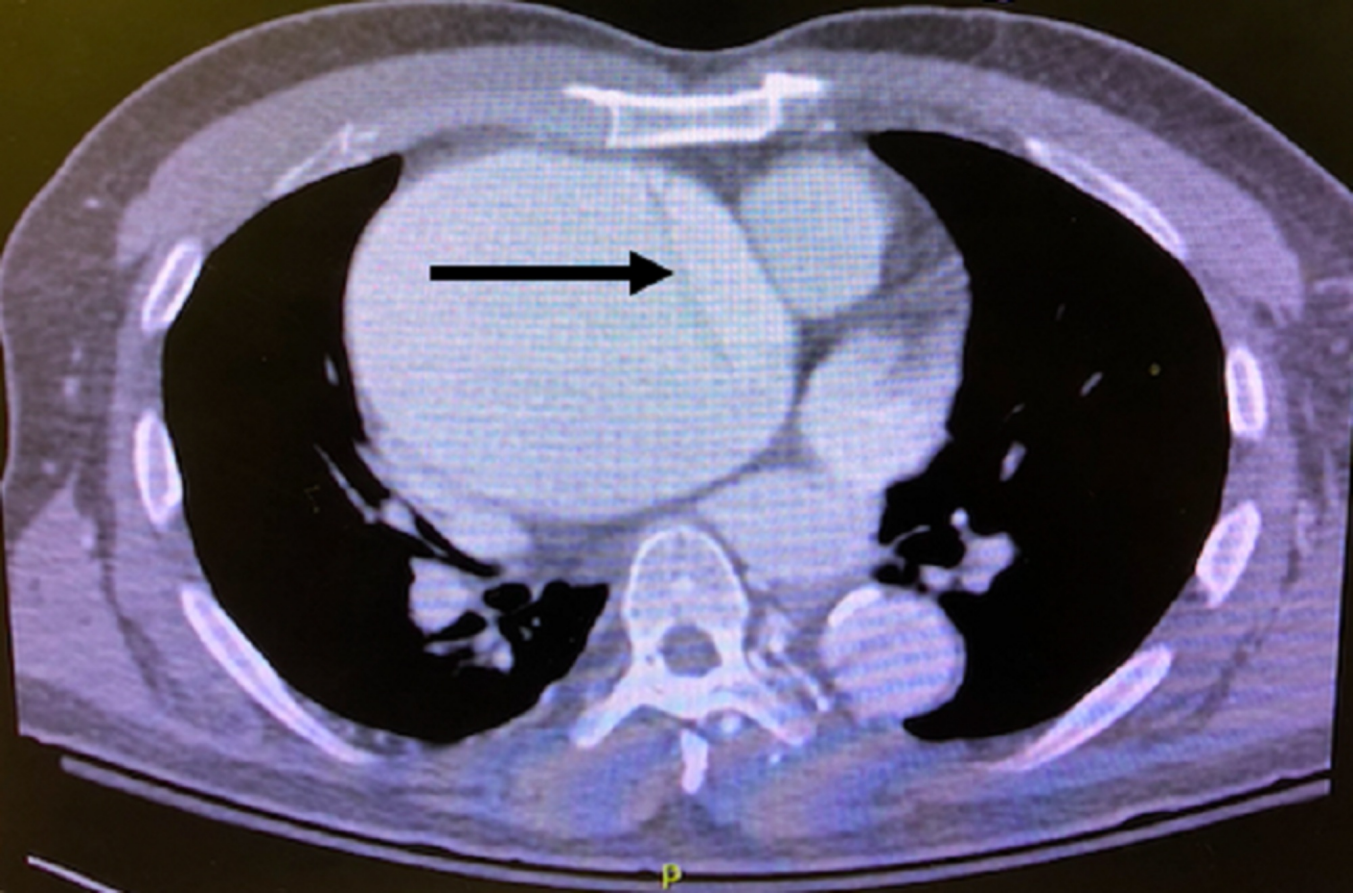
Transverse view of CT angiography of the chest showing a large aortic aneurysm with dissection, with an arrow delineating the dissection flap.
